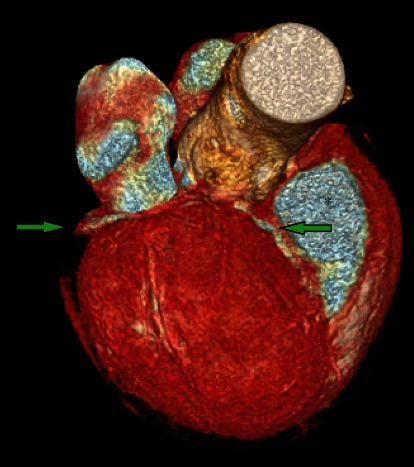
CT coronary showing a large calcified RCA originating posteriorly from the right coronary cusp (green arrow) and a small LAD artery originating from the right coronary cusp into the interventricular groove (green arrow with asterixis).
